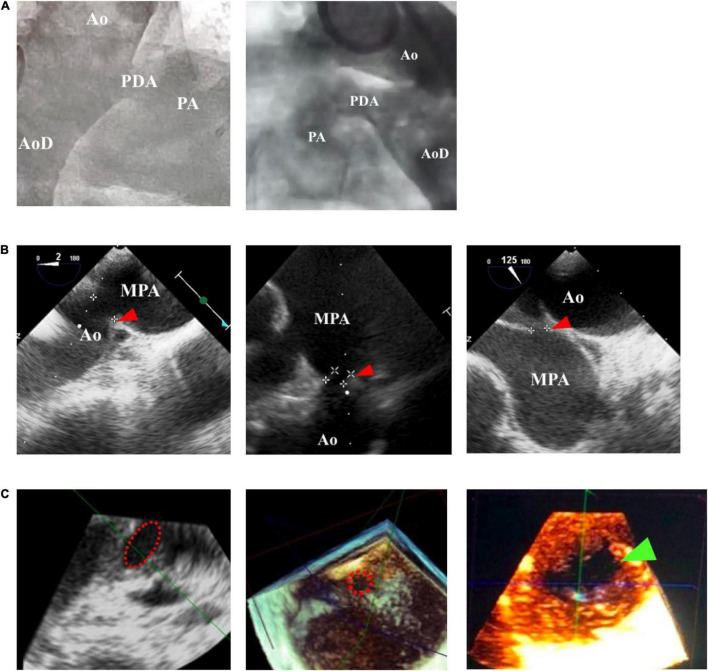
Comparison of patent ductus arteriosus (PDA) size and morphology measurements using fluoroscopy and echocardiography guidance. (A) Fluoroscopy can only provide a 2-dimensional image, whereas (B) transthoracic echocardiography (TTE) and transesophageal echocardiography (TEE) can provide a 3-dimensional structure as shown by the red arrowhead. (C) Cross-sectional PDA morphology measured by using transesophageal echocardiography (TEE), indicated by the dotted red circle. Green arrowhead showed oval-shaped PDA. Ao, aorta; AoD, descending aorta; MPA, main pulmonary artery; PA, pulmonary artery.
